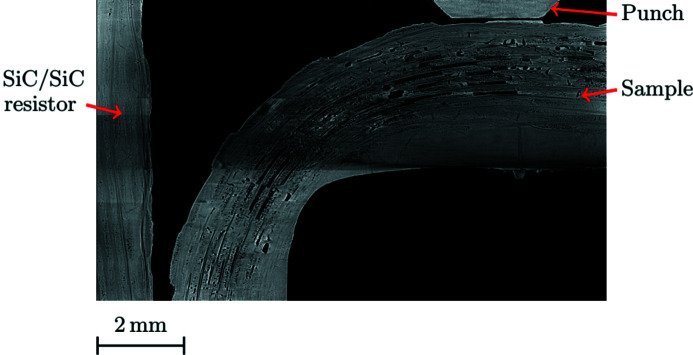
Tomograph of the sample where the beam hardening is noticeable: the part where the length crossed by the X-ray is higher appears darker.
